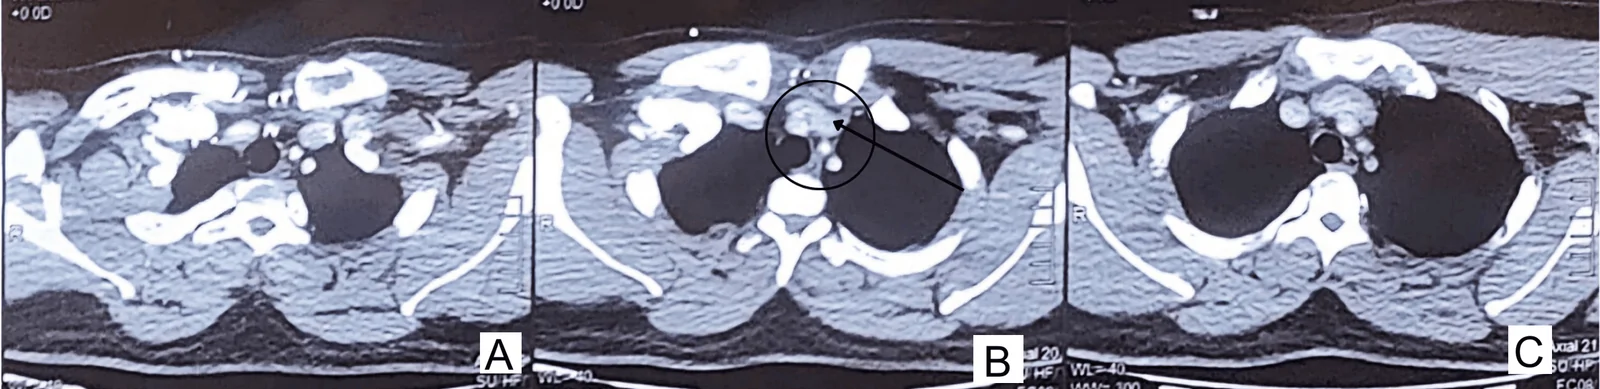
Axial contrast-enhanced CT image of the neck (A): At the level of C6 showing soft tissue thickening along the posterior tracheal wall with mild luminal narrowing; (B) At the level of C7 showing progressive intraluminal mass causing moderate airway compromise; (C): Inferior to C7 showing near-complete luminal obliteration by the posterior tracheal mass
